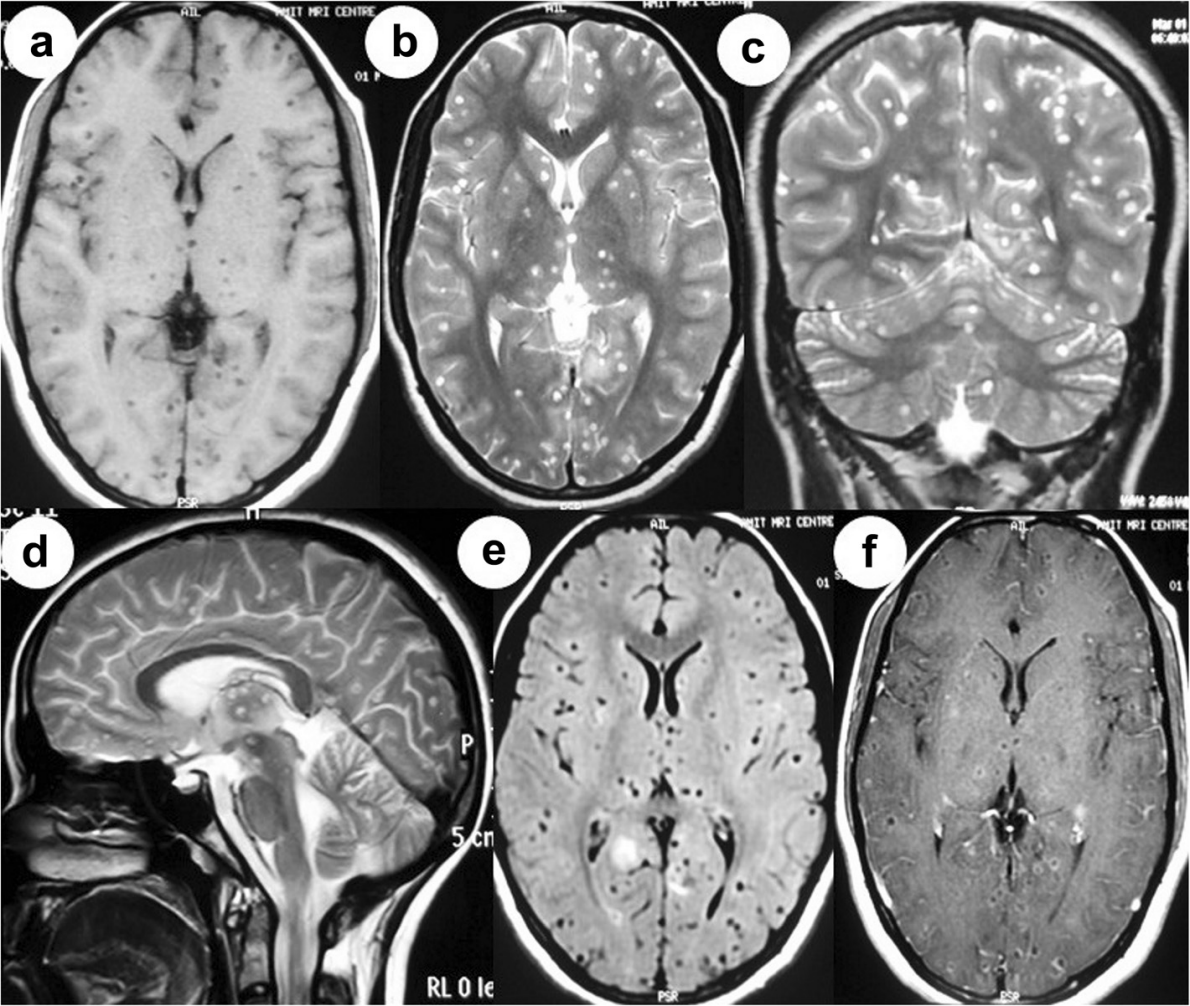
MRI brain showing multiple small cystic lesions in bilateral cerebral hemispheres, brainstem and bilateral cerebellar hemispheres with ring enhancement (a-f).
